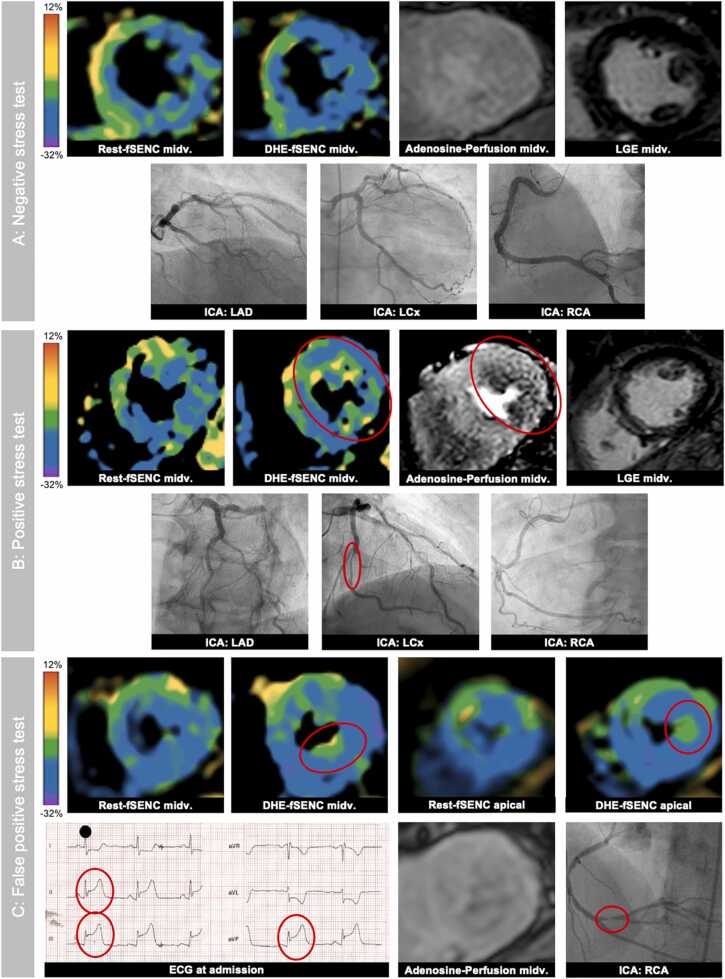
Examples. (A) Negative stress test. 61-year-old, male patient. Progressive dyspnea without typical angina. ICA revealed proximal LAD stenosis with 50% lumen narrowing. Adenosine-perfusion was rated negative. During DHE, the HR increased from 60/min to 98/min, GLS was more pronounced after DHE, from −16.7% to −20.2%. DHE-fSENC was rated negative for ischemia. (B) Positive stress test. 75-year-old, male patient. No dyspnea or typical angina. Former resuscitation during non-ST-elevation myocardial infarction due to LAD stenosis 6 months before. Residual stenosis of LCx (75%). Adenosine-perfusion was rated positive lateral midventricular (2 segments). During DHE HR increased from 54/min to 83/min, GLS slightly increased from −19.0% to −19.5%. In the qualitative analysis of DHE-fSENC a worsening of LS lateral midventricular was observed, similar to adenosine-perfusion – DHE-fSENC was rated positive. (C) “False-positive” stress test. 57-years-old, female patient. Three months before - after progressive, typical angina –PCI including the insertion of a drug-eluting stent into the distal RCA was performed. The patient was referred to stress CMR for residual LCx and LAD stenoses (both 50–75% lumen narrowing). Adenosine-perfusion revealed no significant perfusion deficit. During DHE (HR increase from 75/min to 115/min, GLS increase from −19.6% to −22.5%), a new subendocardial worsening of LS of the inferior wall (2 segments) was detected. Two weeks after CMR the patient presented at chest pain unit with typical angina and ST-segments elevation (II, III, and aVF). In ICA, a subtotal occlusion of RCA, independent of the first lesion, was found and a drug-eluting stent was successfully inserted. *CMR* cardiac magnetic resonance imaging, *DHE* dynamic handgrip exercise, *fSENC* fast Strain-ENCoded imaging, *GLS* global longitudinal strain, *HR* heart rate, *ICA* invasive coronary angiography, *LAD* left anterior descending artery, *LCx* left circumflex artery, *NSTEMI* non-ST-elevation infarction, *PCI* percutaneous coronary intervention, *RCA* right coronary artery
